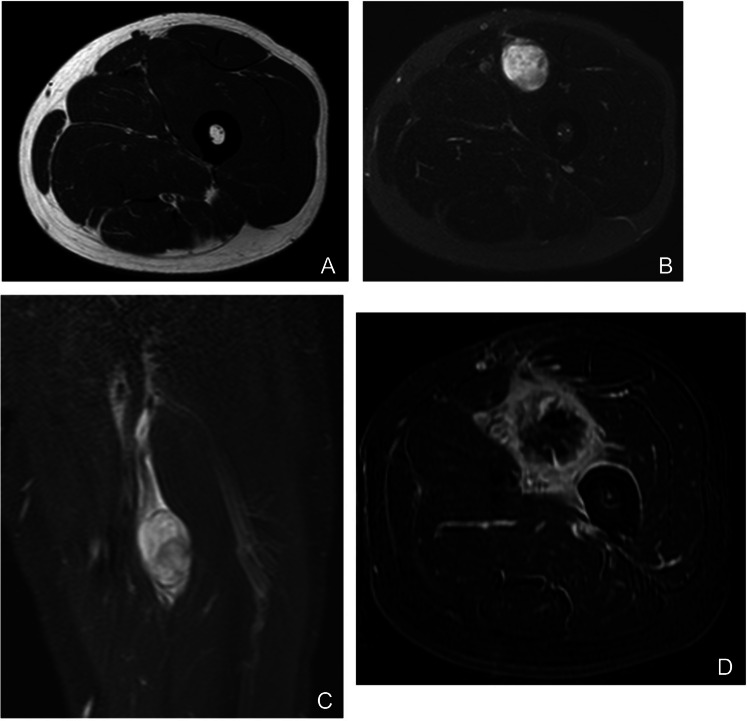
Myxoid pleomorphic liposarcoma of the left thigh. (A) Axial T1-weighted image demonstrates a lobulated intramuscular mass in the anterior compartment, predominantly isointense to skeletal muscle with subtle foci of T1 hyperintensity consistent with a fatty component. (B) Axial fat-suppressed T2-weighted image shows marked heterogeneous hyperintensity. (C) Coronal STIR image delineates the craniocaudal extent of the lesion within the anterior thigh musculature and associated perilesional edema. (D) Axial post-contrast T1-weighted subtraction image demonstrates heterogeneous enhancement of the solid nonlipomatous components, with relative lack of enhancement in the more myxoid regions
